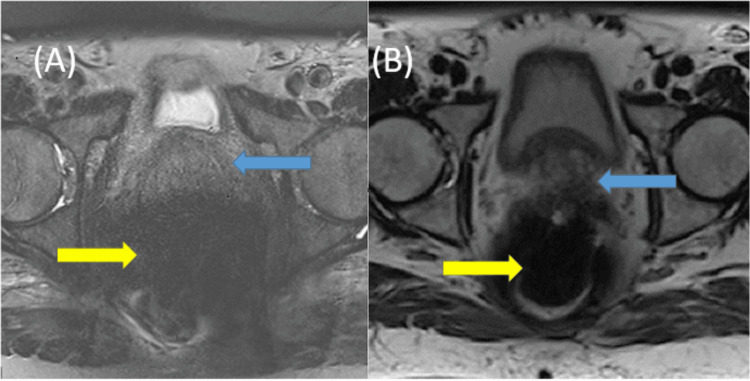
(A) Axial T2 SPACE and (B) axial T2 HASTE MRI sequences showing the prostate (blue arrow) in relation to susceptibility artefact (yellow arrow). SPACE: Sampling Perfection with Application optimised Contrast using different flip angle Evolution; HASTE: Half-Fourier Acquisition single-shot Turbo spin Echo imaging; MRI: magnetic resonance imaging
